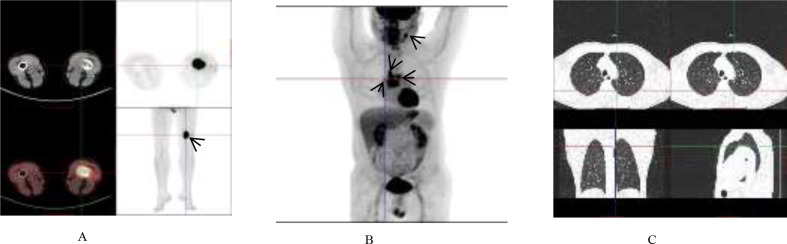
Summary of case l PET/CT images. (A) Metastatic lesion in the distal left femur. (B) Metastatic lesions in the mediastinal lymph nodes (regions 2R, 4R, and 4L) and the left para-carotid lymph node; (C) No significant increase in radiotracer uptake was observed in either lung.
